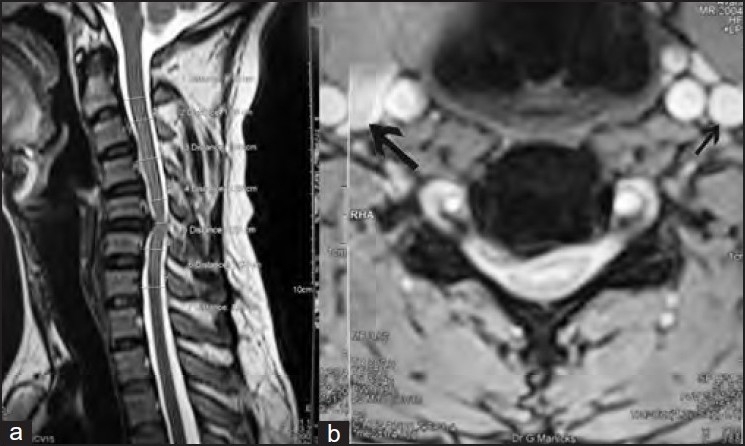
T2T1W sagittal MRI section of cervical spine (a) and horizontal cut section at C5C6 level (b) with dilated right internal jugular vein (long arrow) in comparison with left internal jugular vein of normal caliber (short arrow)
